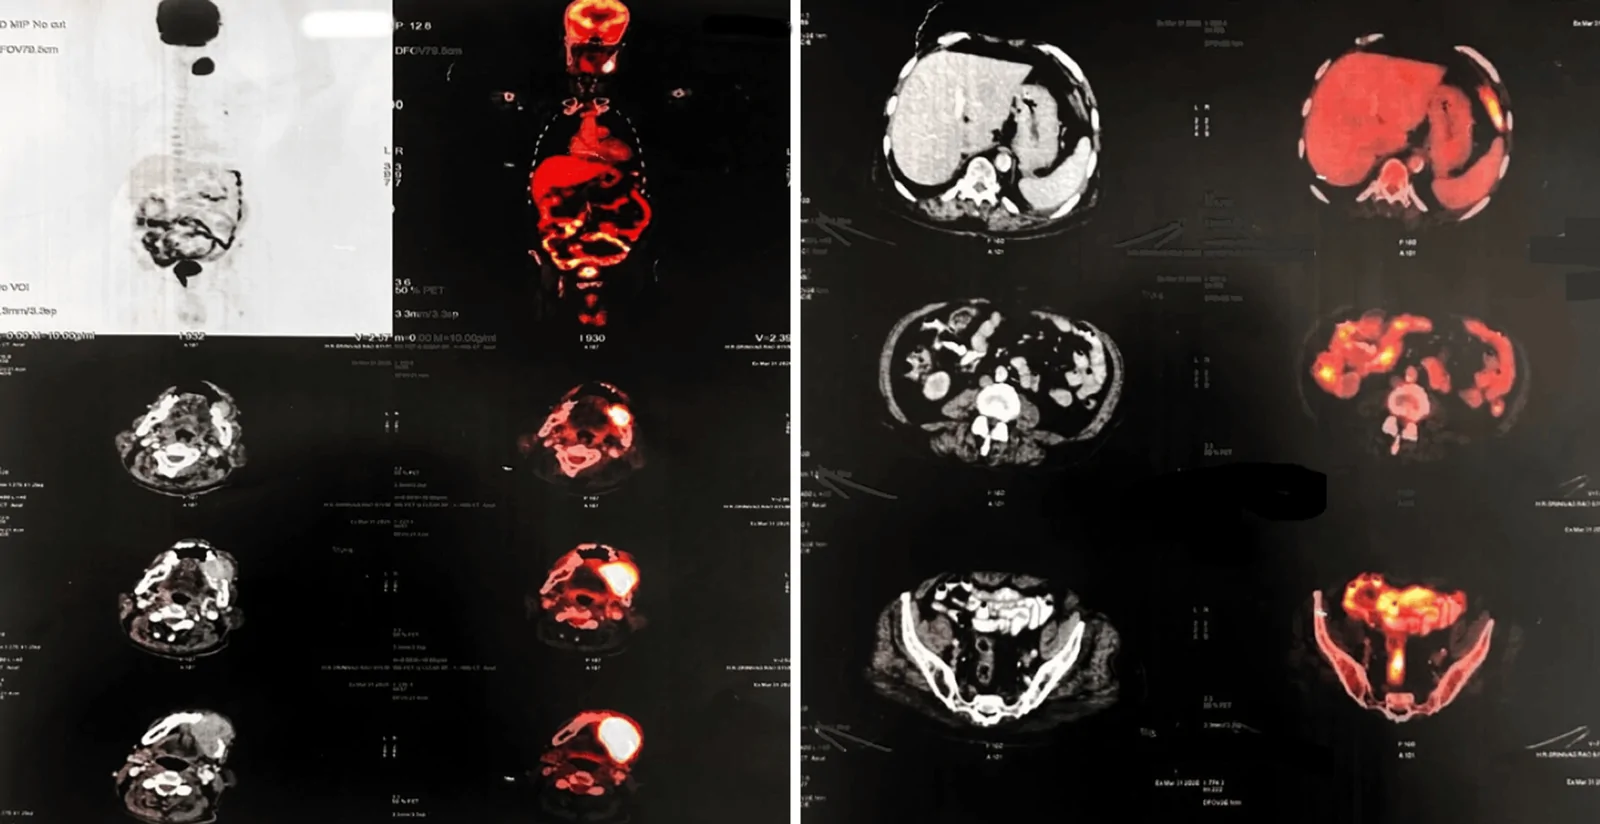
18F-FDG PET-CT findings. (a) Whole-body MIP and fused PET-CT images showing an intensely FDG-avid destructive soft tissue mass involving the left hemimandible. (b) Additional FDG-avid osteolytic lesions involving the left fourth rib, left iliac bone, and multiple vertebral bodies, confirming multifocal skeletal disease compatible with multiple myeloma. 18F-FDG PET-CT, 18F-fluorodeoxyglucose positron emission tomography-computed tomography; MIP, maximum intensity projection
